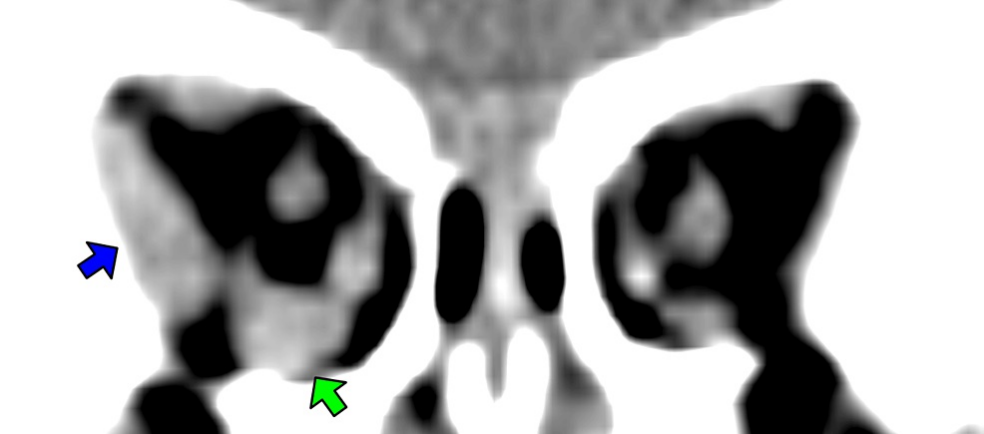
CAT scan image at the first visit. A coronal view of the CT orbit revealed enlargement of the lateral rectus and inferior rectus. The blue arrow is the right lateral rectus muscle, and the green arrow is the right inferior rectus muscle. CT, computerized tomography; CAT, computerized axial tomography
